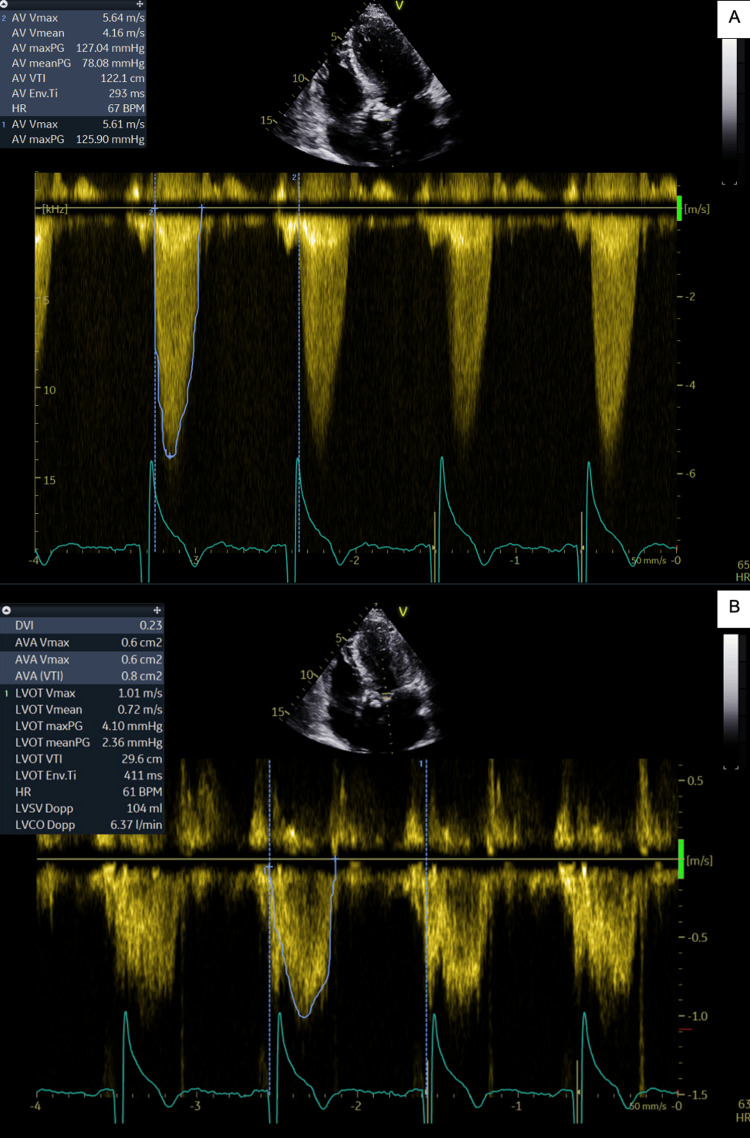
Pre-TAVI echocardiogram showing signs of severe AS (A) Continuous-wave Doppler demonstrating severe AS with an aortic valve Vmax of 5.67 m/s (severe >4 m/s). (B) Pulsed-wave Doppler of the left ventricular outflow tract, used in the continuity equation to calculate a valve area of 0.6 cm² (severe <1 cm²) [6]. AS, aortic stenosis; TAVI, transcatheter aortic valve implantation
